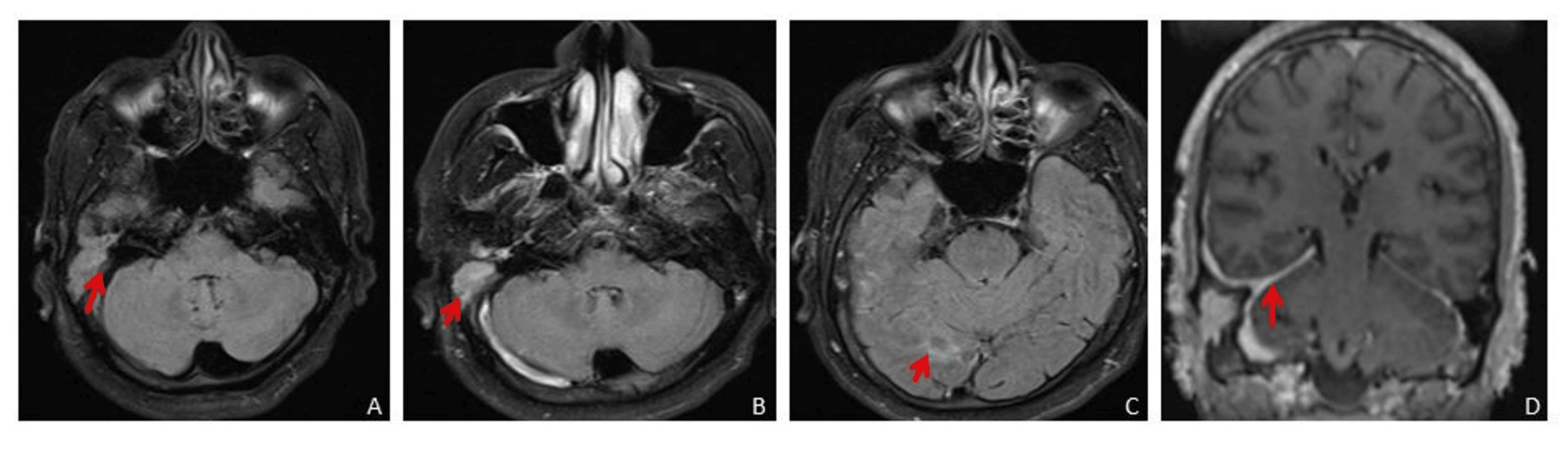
(A) Axial FLAIR (plain) showing slightly hyperintense soft tissue intensity lesion in the right middle ear cavity and mastoid air cells. (B) Axial post-contrast FLAIR showing enhancing lesion in the right middle ear cavity and mastoid air cells. (C) Axial post-contrast FLAIR showing leptomeningeal enhancement in sulcal spaces in the right temporal and occipital region. (D) Coronal post-contrast T1FS showing thickening and dense enhancement of the right leaf of tentorium cerebelli, pachymeningeal enhancement in the right temporal region, enhancing lesion in the right middle ear cavity and mastoid air cells with erosion of the outer mastoid cortex. FLAIR: Fluid-attenuated inversion recovery; T1FS: T1-weighted fat-saturated
